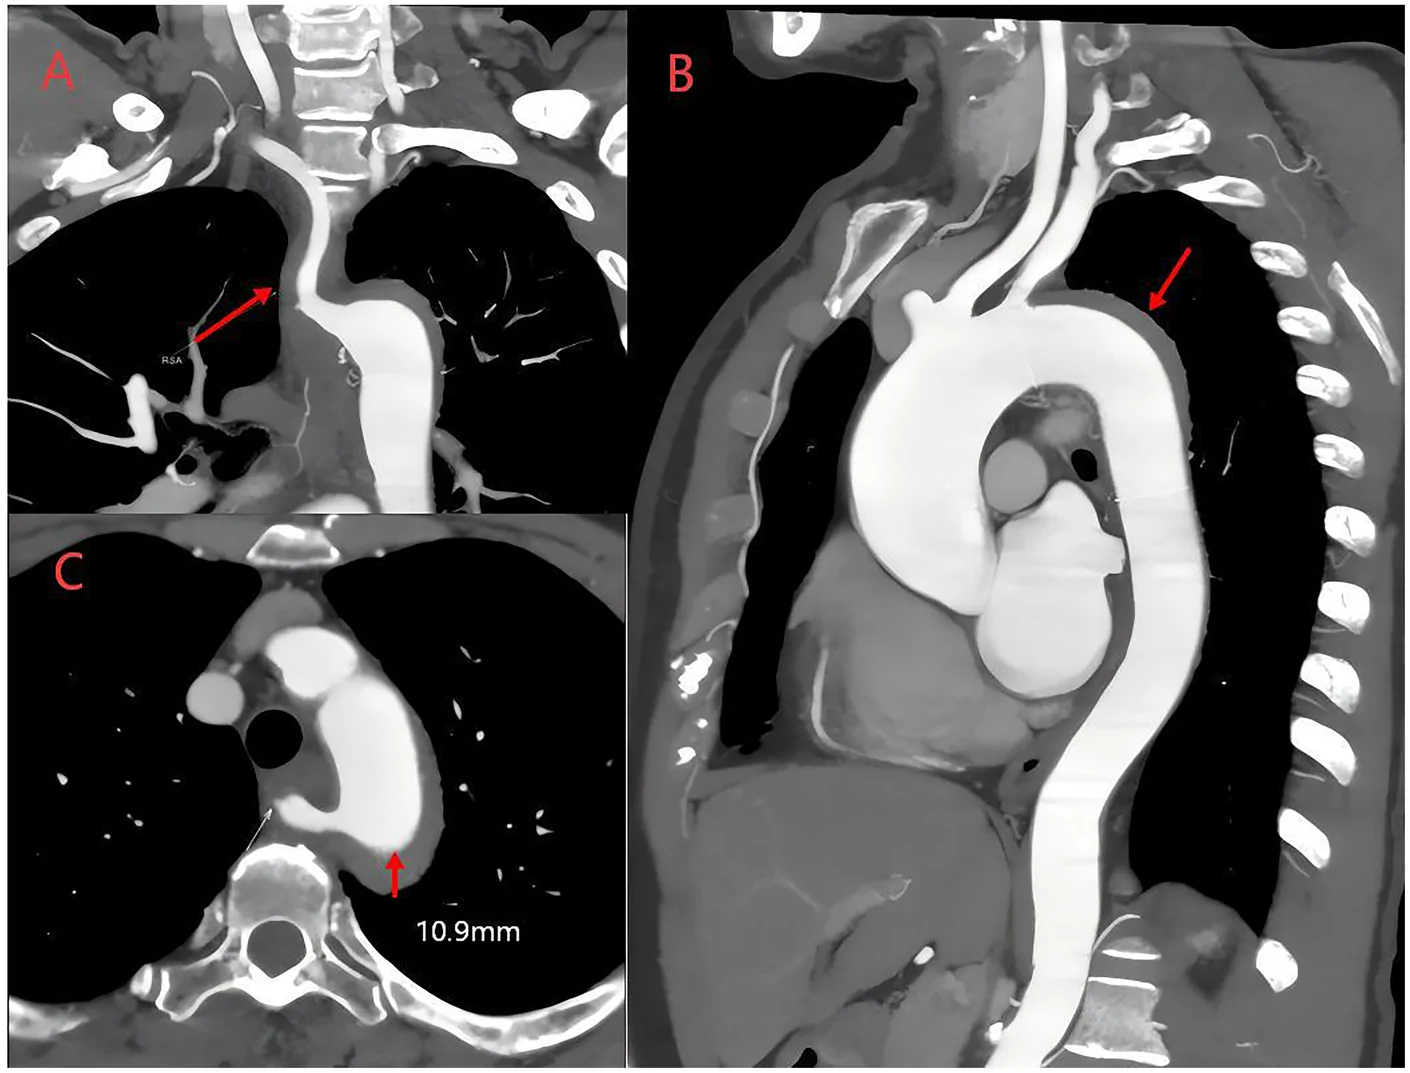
Follow-up CTA images. (A) Complete thrombosis of the false lumen in the distal aortic arch, descending aorta, aberrant right subclavian artery (arrow), and right axillary artery. (B) Marked reduction in the size of the false lumen (arrow). (C) The false lumen has a maximum width of approximately 10.9 mm (arrow).
